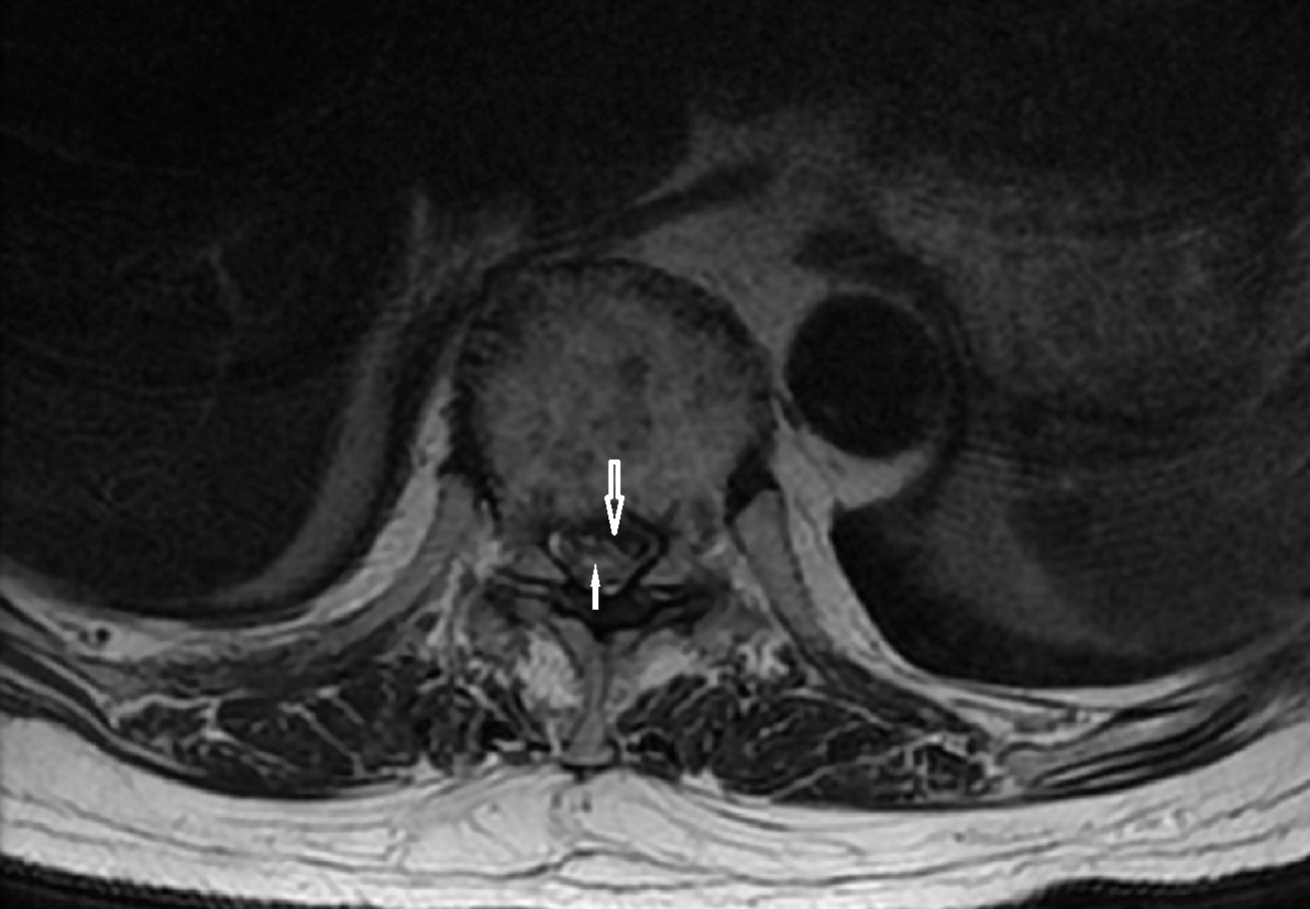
MRI Axial Thoracic Spine T2 Weighted Unfilled arrow shows hematoma. Filled arrow shows edema in the thoracic spinal cord.
